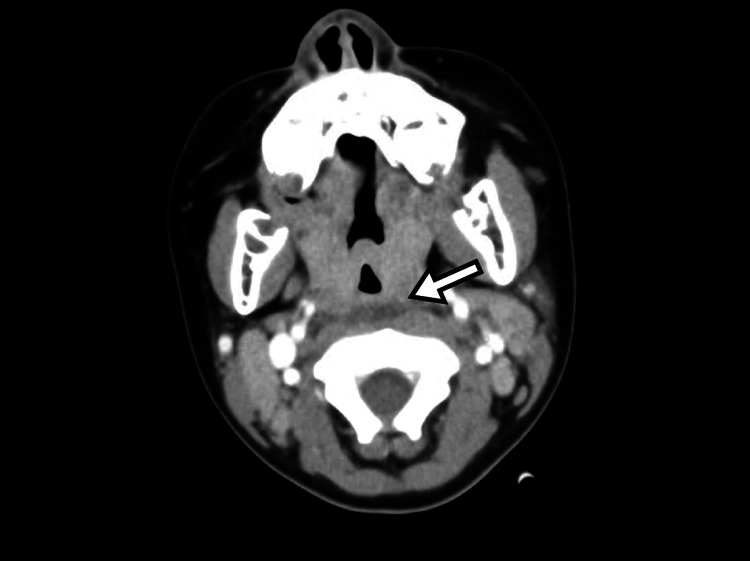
Retropharyngeal edema without abscess formation. Contrast-enhanced CT shows retropharyngeal edema without ring enhancement, low-attenuation fluid collection, intralesional gas, or any drainable collection. The arrow indicates the retropharyngeal edema.
